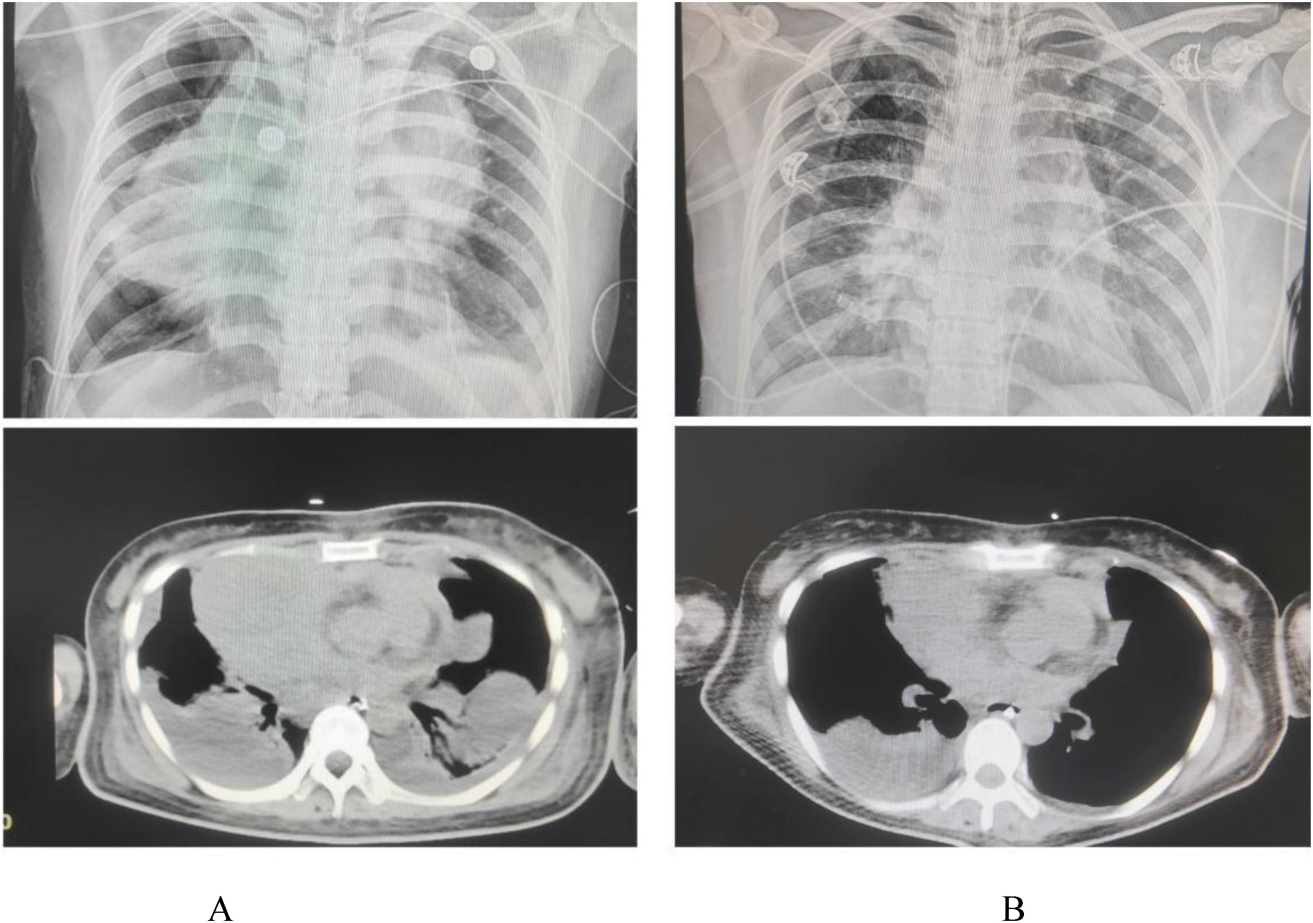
(A) Shows the patient’s initial CT scan and X-ray upon admission, while (B) displays the CT scan and X-ray taken 15 days later.
